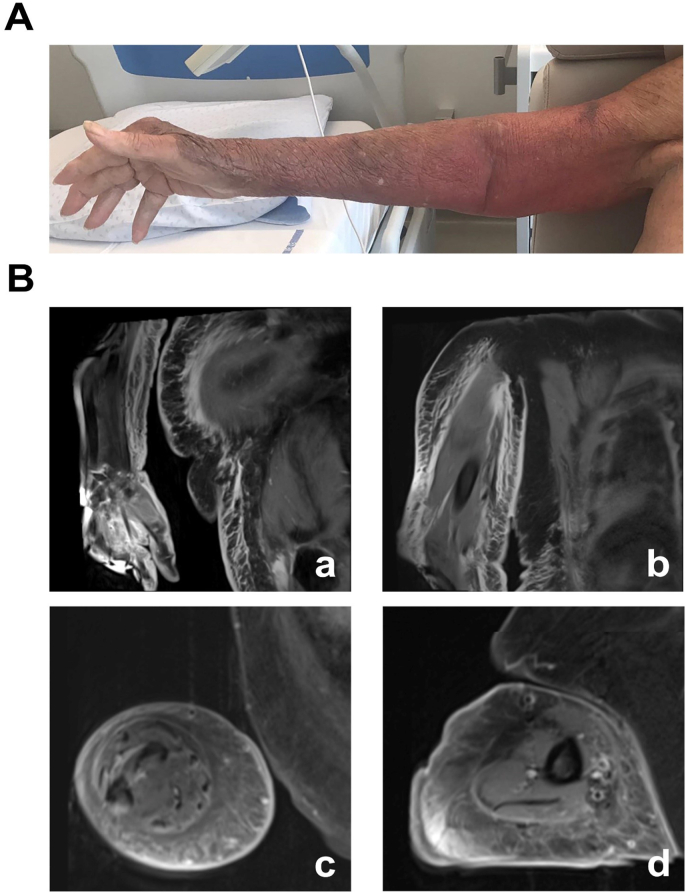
A. Erythematous lesion of the inner side of the upper arm. B. MRI images showing diffuse dermo-hypodermitis of the right arm and forearm associated to superficial fasciitis of the *biceps* and *triceps brachialis* as well as of *extensor* and *flexor carpi ulnaris*.
